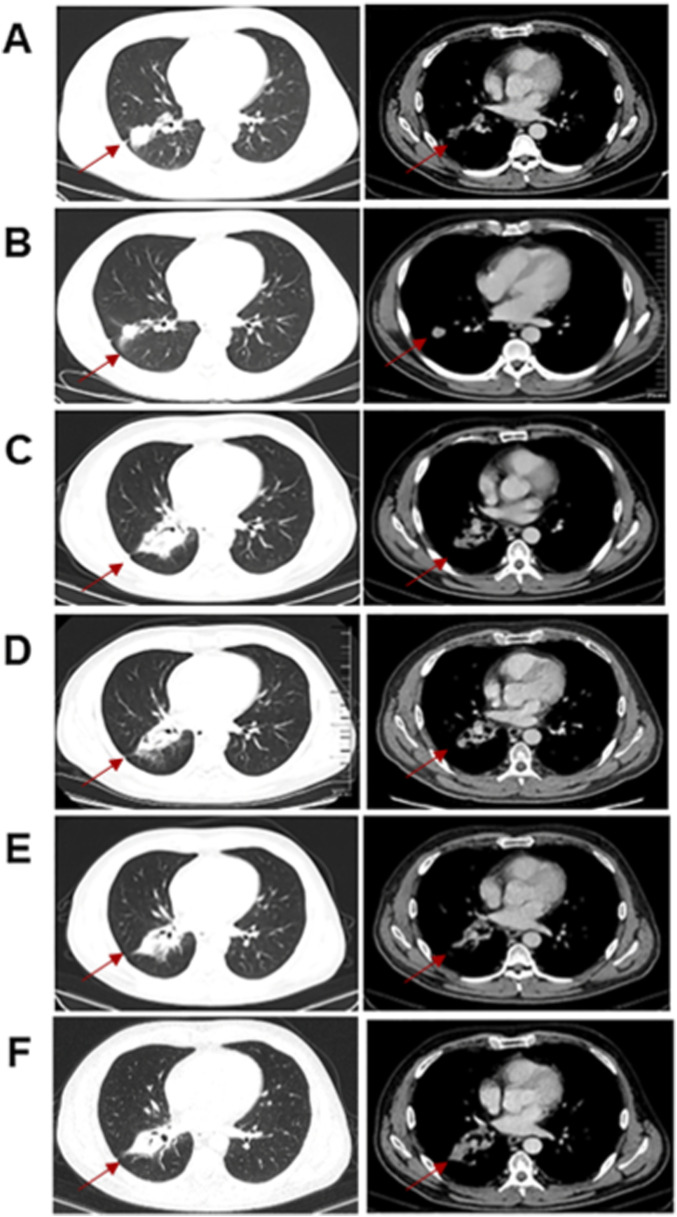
(A) Computed tomography (CT) scan of thorax before initiation of concurrent chemoradiotherapy. (B) CT scan of thorax after chemoradiotherapy. (C) CT scan of thorax before afatinib therapy. (D) CT scan of thorax after 1 month of afatinib therapy. (E) CT scan of thorax before osimertinib. (F) CT scan of thorax after 2 months of osimertinib.
